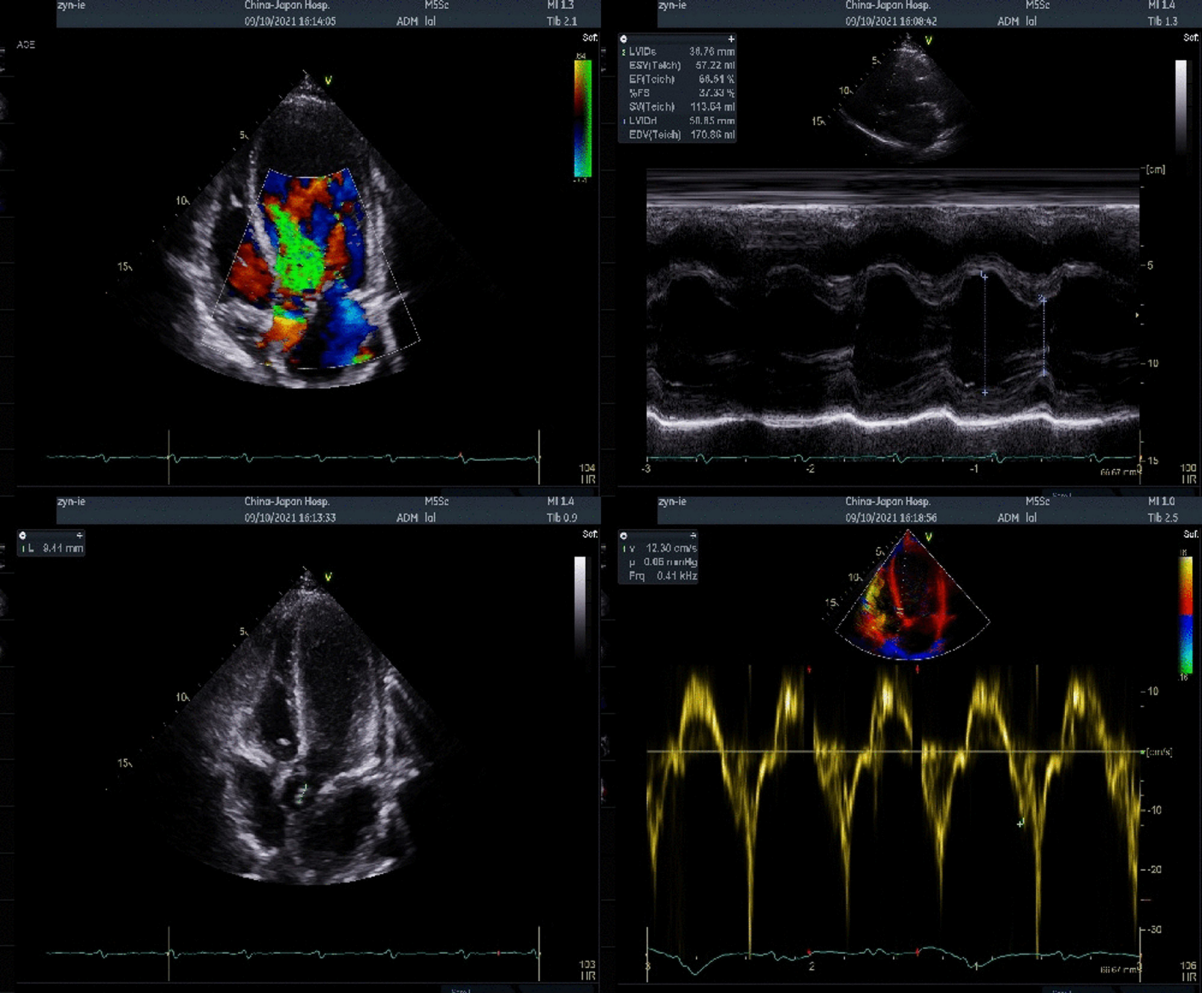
Transthoracic echocardiography (including tissue Doppler) showing aortic valve bivalve malformation, abnormal echo, and severe regurgitation left atrial dilatation, and moderate mitral regurgitation, with LVEF of 66%.
